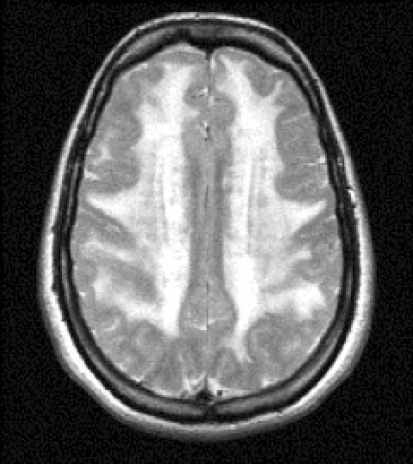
A typical pattern of distribution of white matter hyperintensities in a T2 MRI of a 42-year-old woman affected by CADASIL
